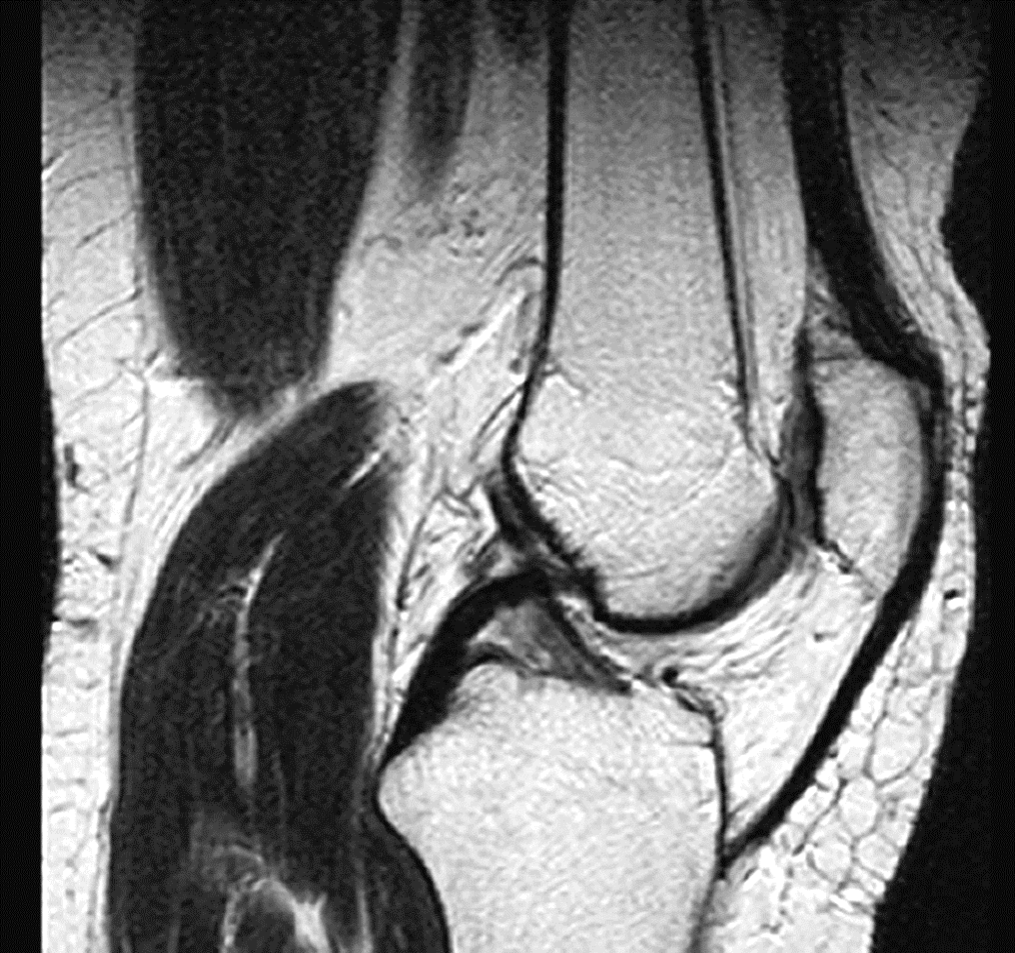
In magnetic resonance image, patellar surface lesion is interpreted to be grade II
